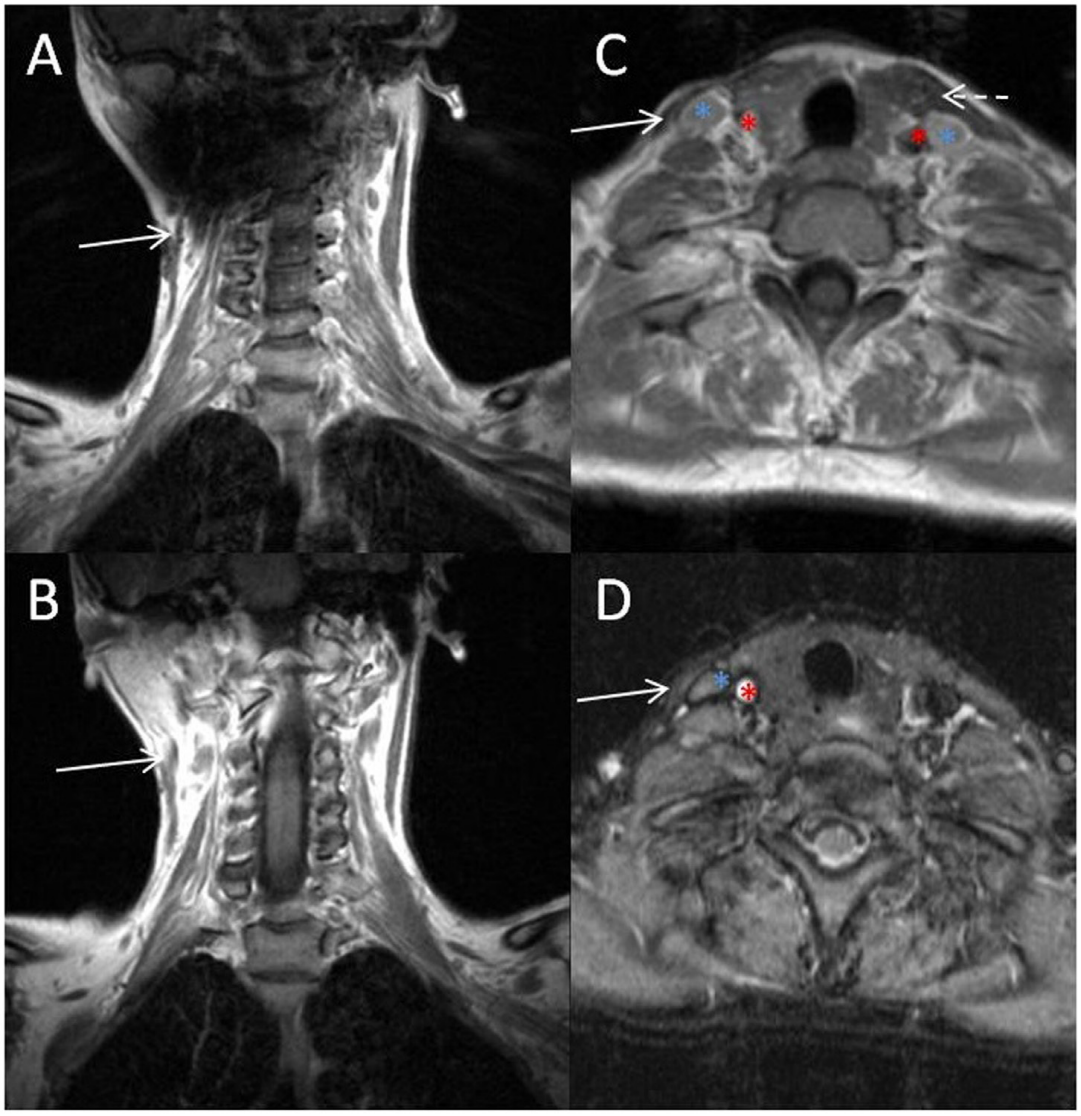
MRI of fibrotic band and remnant SCM. Sequential coronal (A,B) and axial (C,D) images of the fibrotic band (solid arrow) on coronal sequences, remnant SCM (solid arrow) on axial images and the carotid artery (red asterisk), internal jugular vein (blue asterisk), and left SCM (dashed arrow) are indicated for reference.
